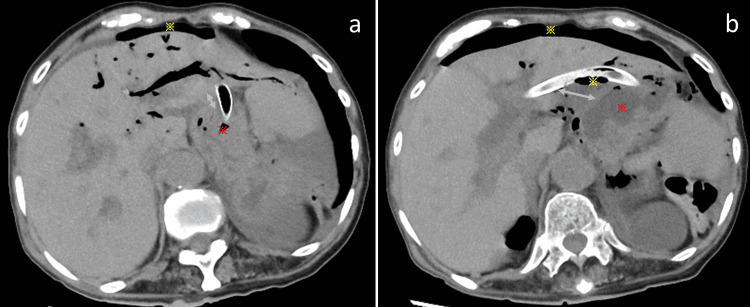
Postoperative images a: computed tomography on the following day reveals the presence of free air (yellow asterisk), although the liver and stomach (red asterisk) remain closely attached (double blue arrow); b: computed tomography performed three days later demonstrates an increase in free air (yellow asterisk) with a greater distance (double blue arrow) between the liver and stomach (red asterisk).
